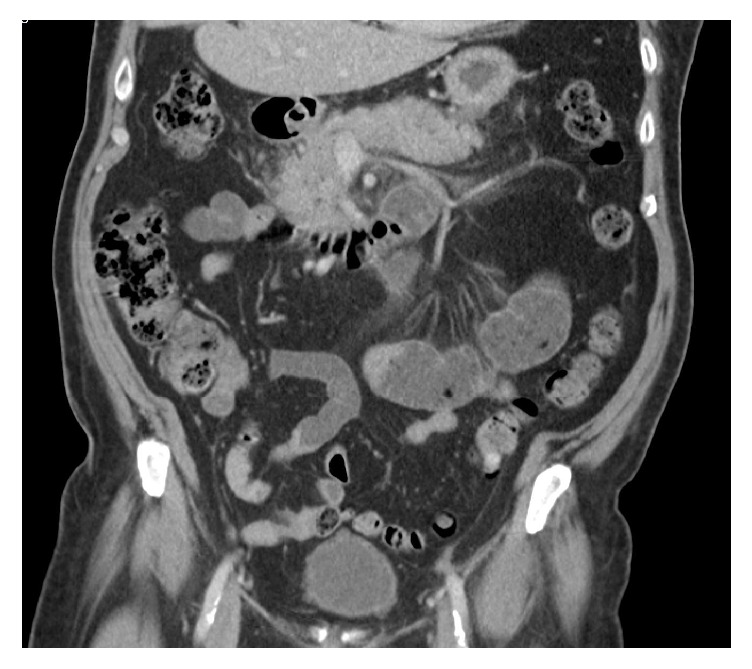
Coronal contrast enhanced CT image showing a homogenously enhancing bulky pancreas with peripancreatic haziness (arrows) with no evidence of collections consistent with noncomplicated acute interstitial pancreatitis.
